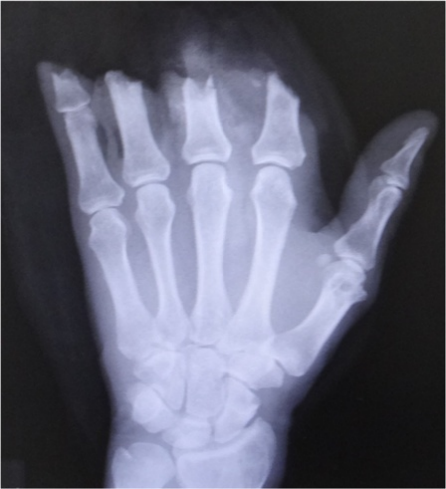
Case 1. An X-ray image of injured hand
